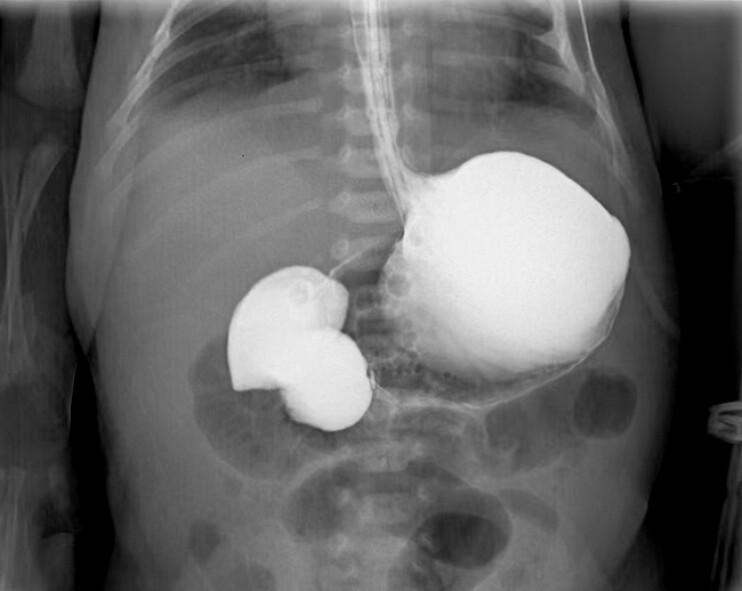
Upper gastrointestinal radiography confirmed the complete obstruction of the duodenum.
